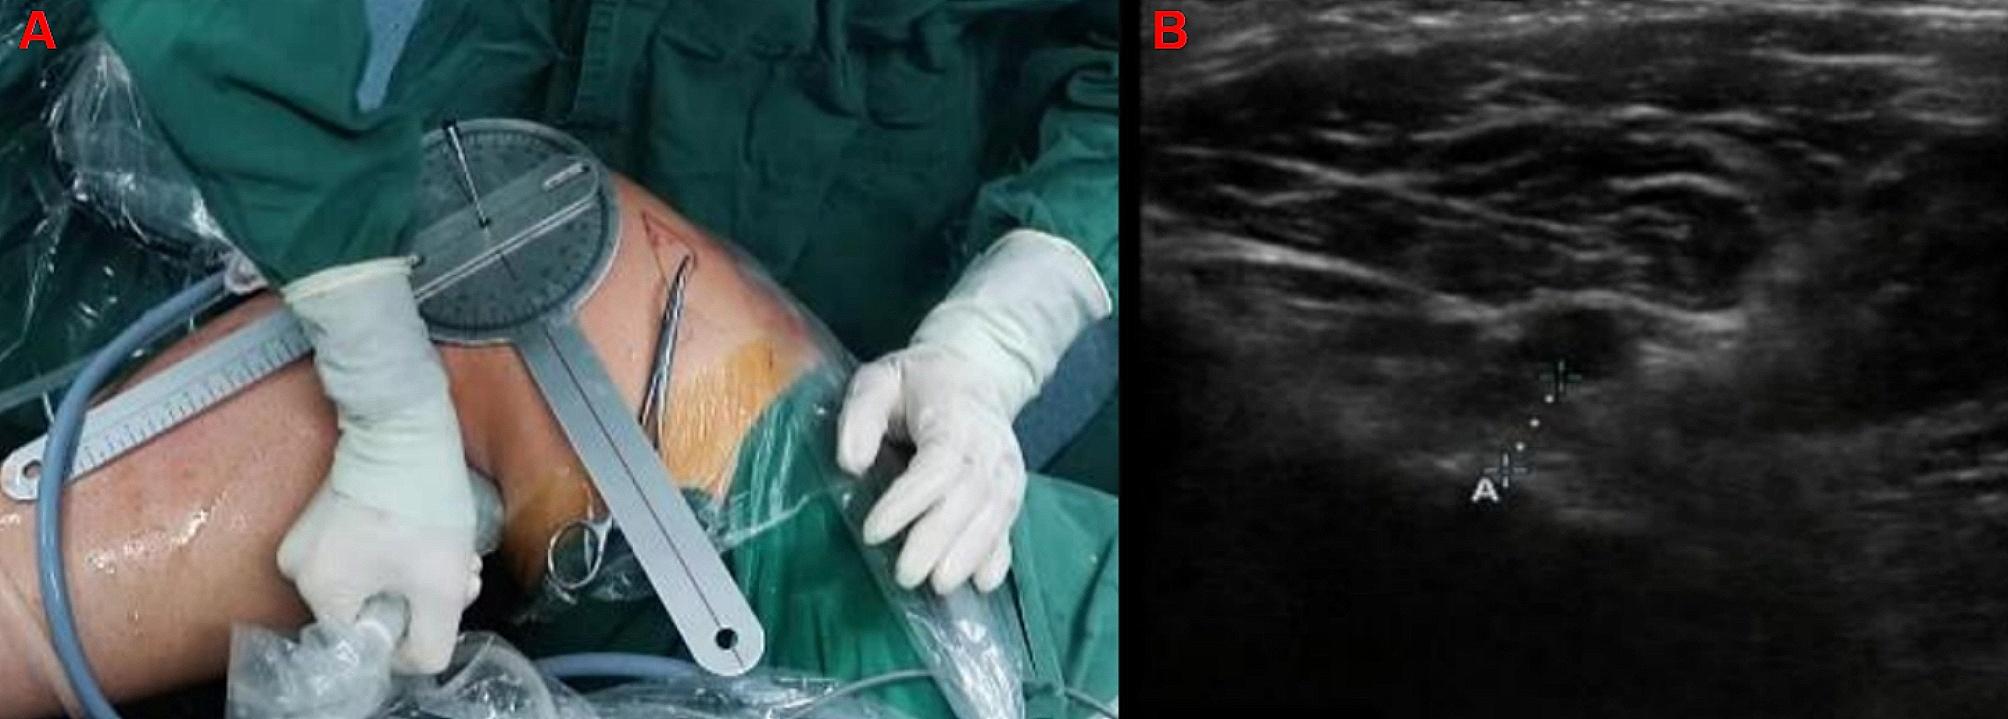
Ultrasound measures the distance between the posterior edge of the tibial plateau bone cortex and the popliteal artery at different flexion angles of the knee. 3A: Measure the distance between at knee flexion 90; 3B: Ultrasound measures the distance between the posterior edge of the tibial plateau bone cortex and the popliteal artery)
